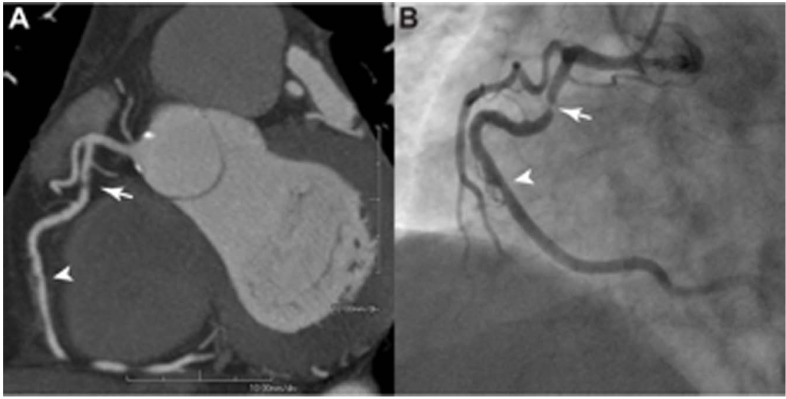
Cardiac computed tomography study and invasive coronary angiography on the same patient. A: Multiplanar reformatted image
